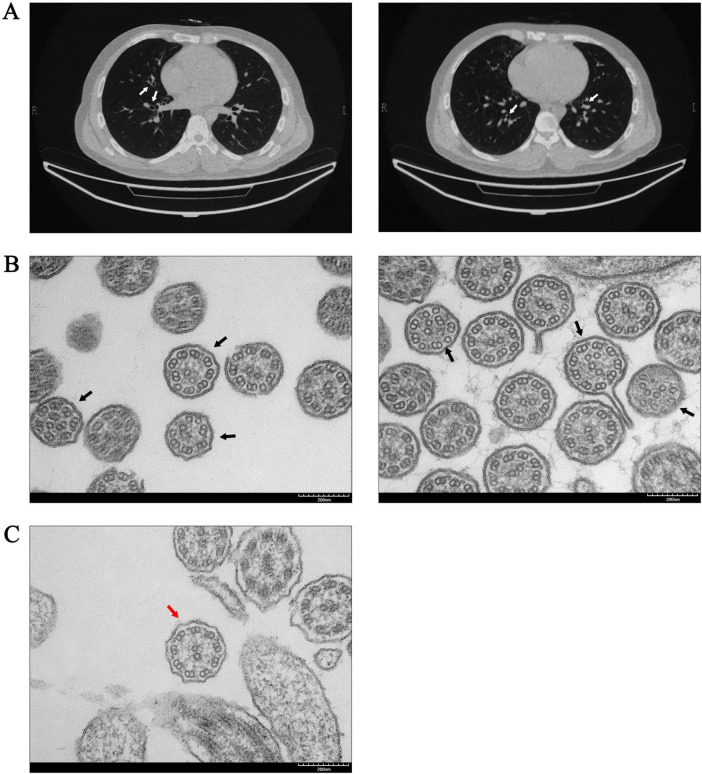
Abnormal manifestations in the respiratory system of the patient. (A) The chest computed tomography of the patient indicated bronchiectasis (white arrows). (B) TEM of the patient’s bronchial mucosa revealed abnormalities in the “9 + 2” microtubule structures. The central microtubules and peripheral doublet microtubules exhibited disordered arrangements, along with structural or numerical abnormalities (black arrows). (C) “9 + 2” ultrastructural arrangement of bronchial mucosa in a non-PCD control was normal (red arrow).
